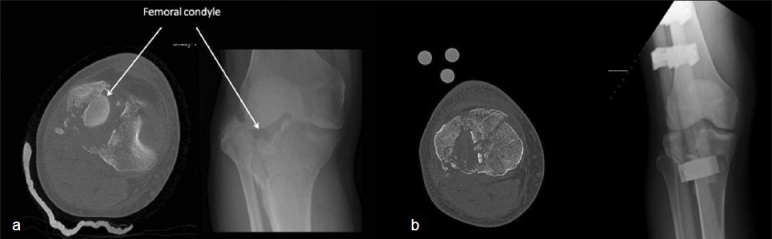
(a) CT scan before spanning external fixation - note the difficulty in interpretation of the CT due to overlapping femoral condyles. (b) CT scan after spanning external fixation - tibia is out to length and femoral condyle does not interfere with the interpretation of fracture configuration
